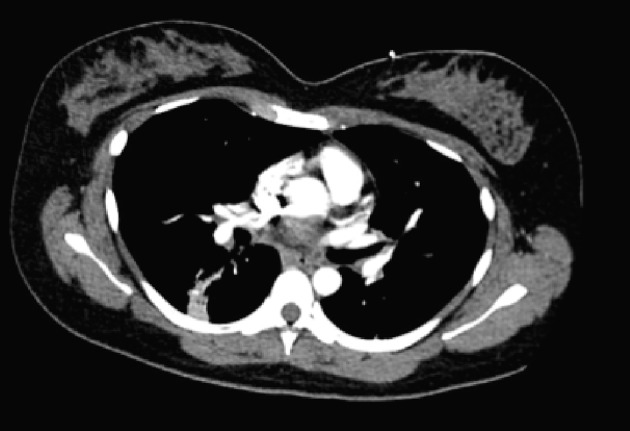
CTA chest: tree-in bud changes in right lower lobe without clear signs of emphysema.
